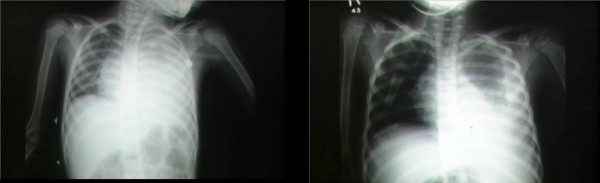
Chest roentgenogram of child with thoracic empyema on hospital days 1 (1A) and 6 (1B).
